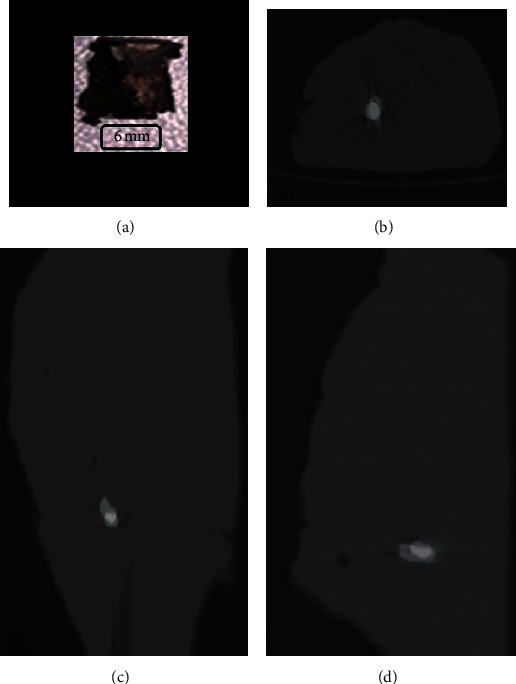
Shrapnel *B* (a). Fused images before and after MRI exposure in axial (b), coronal (c), and sagittal (d) plans.
